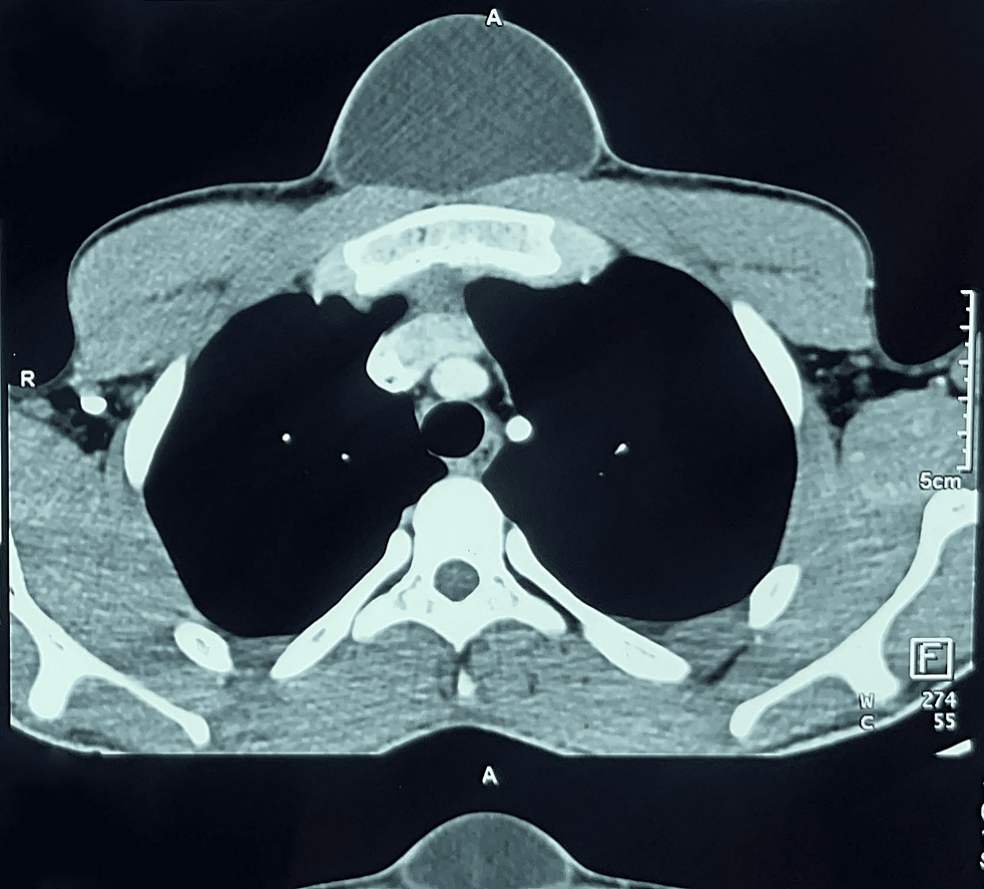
CT scan showing the pre-sternal mass (axial section)
